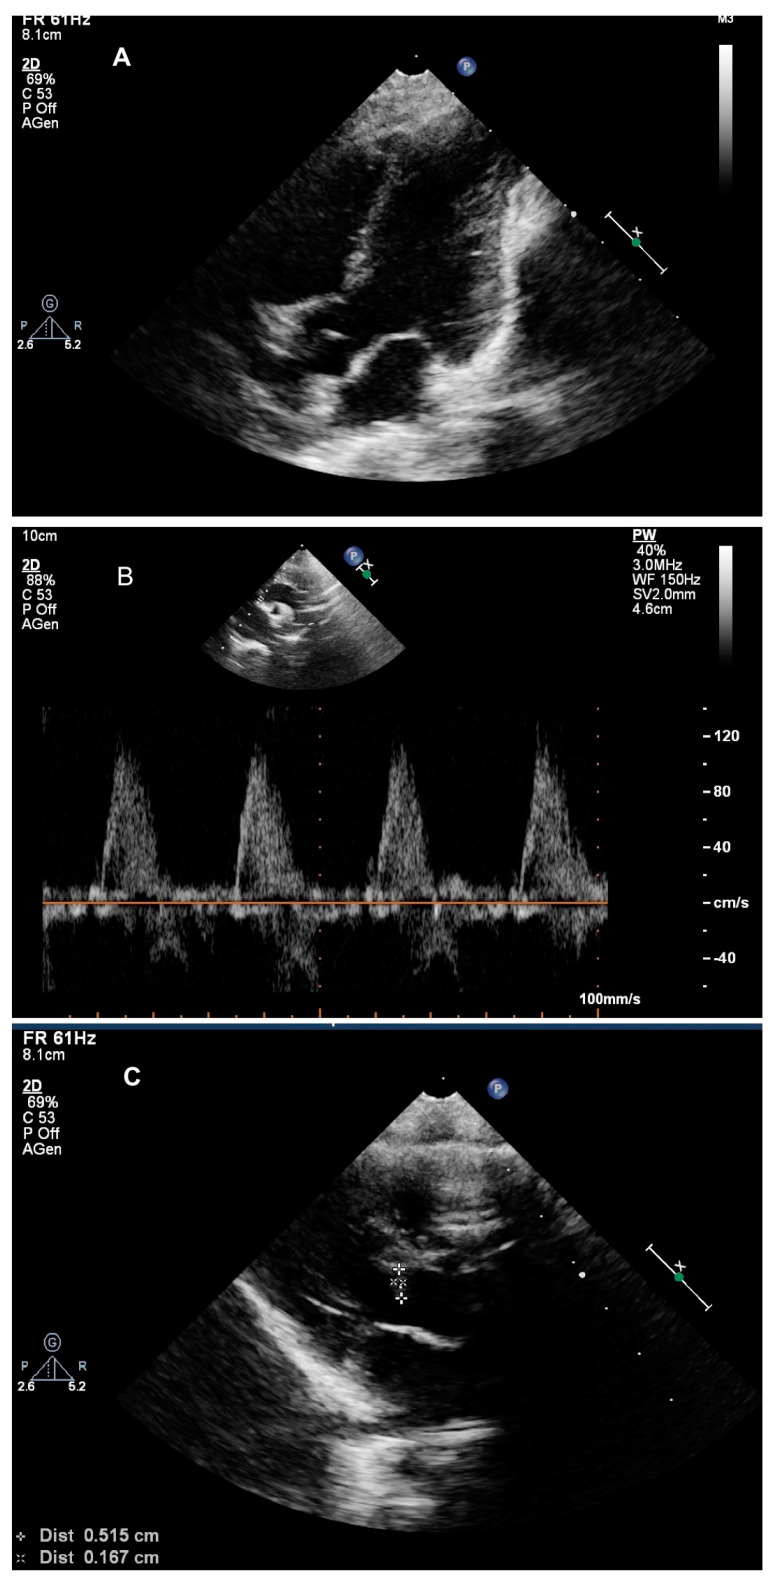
Echocardiogram after 1 week of Everolimus treatment. Panel (A): Disappearance of the left ventricle rhabdomyomas; Panel (B): Normal transaortic gradient; Panel (C): Marked reduction of the subaortic mass.
